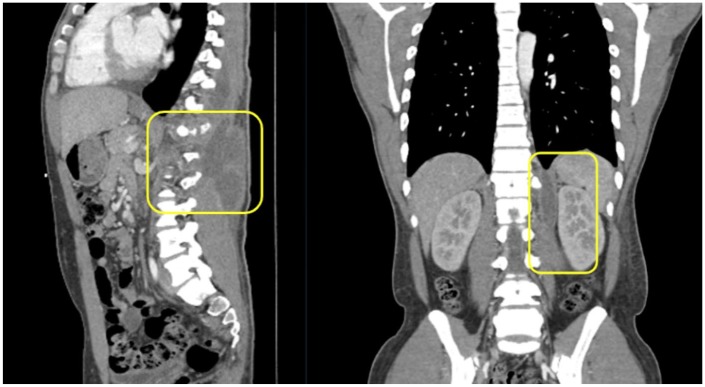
Thoraco-abdominal computed tomography scan showing T7-T12 paraspinal
